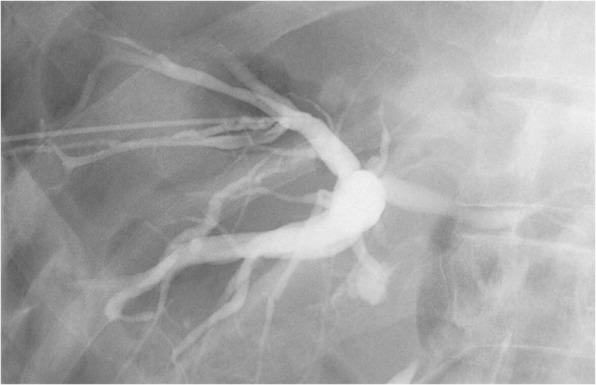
Percutaneous transhepatic biliary drainage contrast radiography. Only bile ducts in the posterior segment were visualized without a bile leakage
